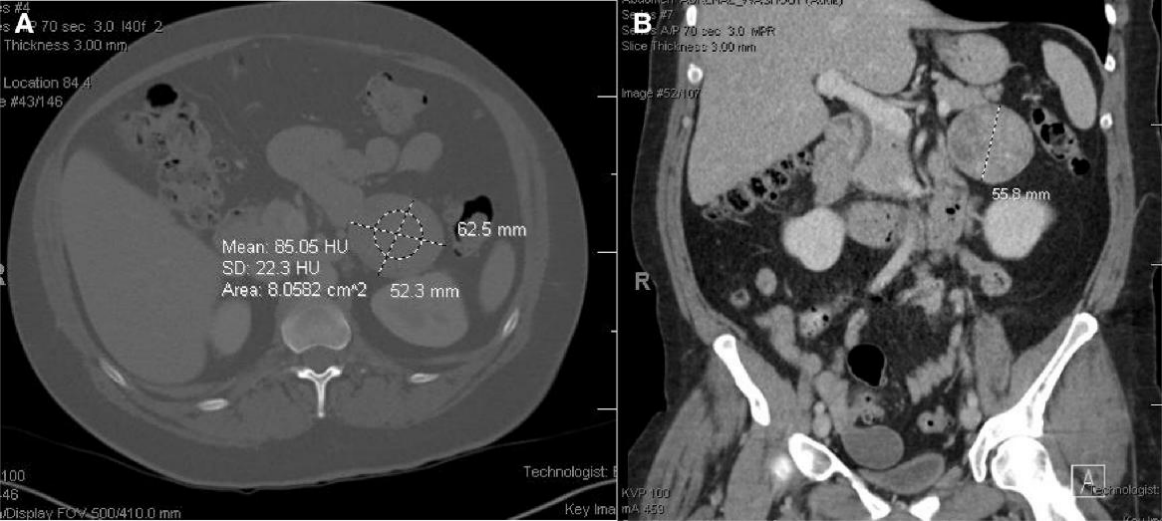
Axial (A) and coronal (B) computed tomography of the abdomen with IV contrast demonstrating left adrenal mass. The study revealed a 6.3 × 5.2 × 5.6 cm left adrenal mass. It measured 22 Hounsfield units (HU) on noncontrast imaging, 85 HU on 70-second imaging, and 48 HU on 15-minute delayed phase. Calculated relative washout was 43.5% and absolute washout was 59.4%. Additionally, there was another 1.1-cm low-density nodule in the on left adrenal genu. The right adrenal gland also had a 1.2-cm low-density nodule with 0 HU on all phases compatible with a lipid-rich adenoma.
